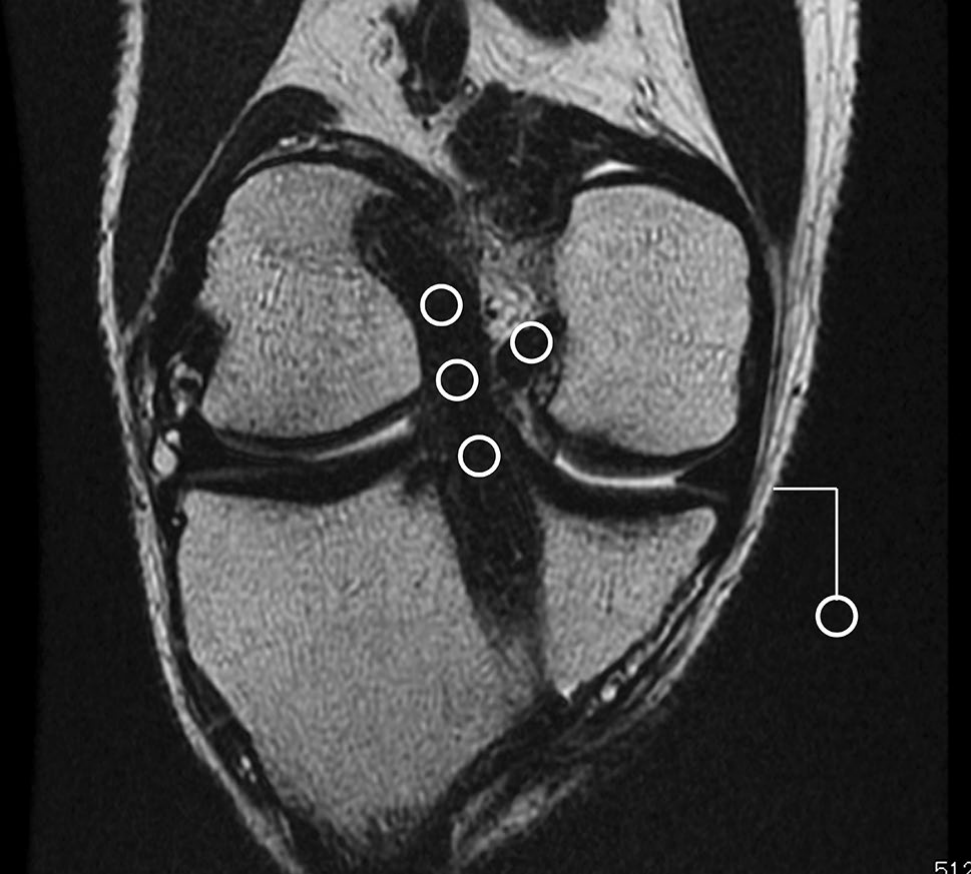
T2-weighted magnetic resonance image of the transplanted graft: oblique coronal slice. Graft signals at the 5 points (white circles) are calculated with circular regions of interest: three intra-articular sites of transplanted graft (proximal, mid-substance, and distal), posterior cruciate ligament, and background.
